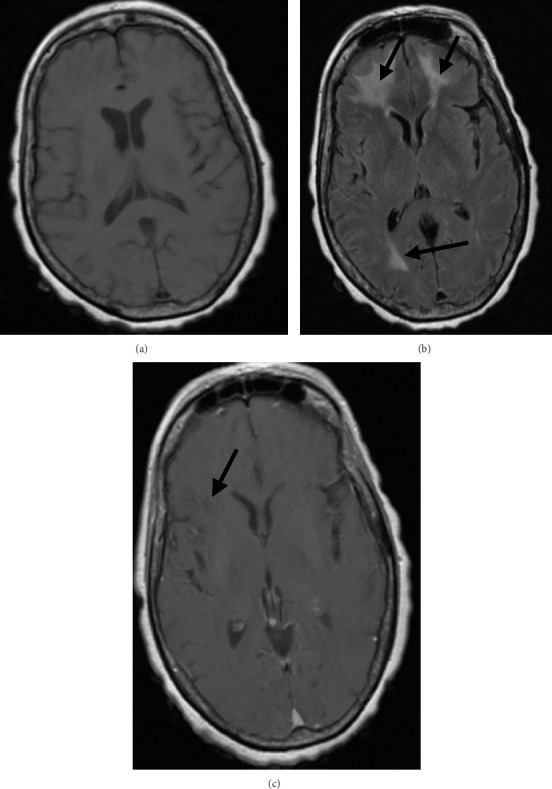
MRI brain, with and without contrast—hospital admission #1. Abnormal findings are indicated by the arrows. (a) Axial T1—no significant findings. (b) Axial T2 FLAIR-increased signal abnormality in the bilateral frontal and right occipital lobes. (c) Axial T1 postcontrast—faint linear enhancement of the white matter parenchyma in the bilateral frontal lobes (greater on the right). Altogether, this MRI study demonstrates diffuse areas of white matter enhancement, vasogenic edema, and diffusion restriction consistent with a cerebral vasculitis within the parietal and frontal lobes.
